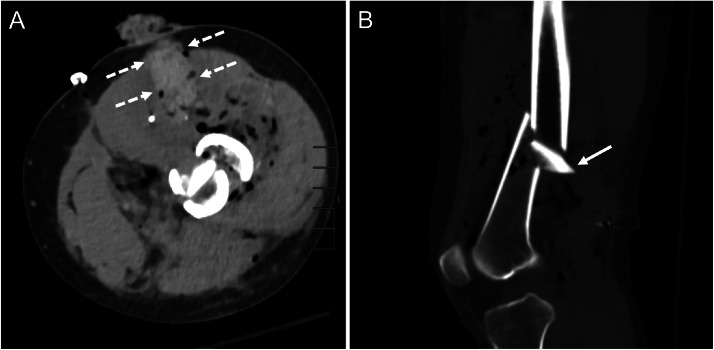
Axial (A) and sagittal (B) CT images of the left distal femur at initial presentation demonstrate the anteromedial to posterolateral trajectory (dashed arrows) of the projectile with extensive associated subcutaneous emphysema and a displaced comminuted distal femoral fracture with a posteriorly directed sharp bony fragment (arrow).
